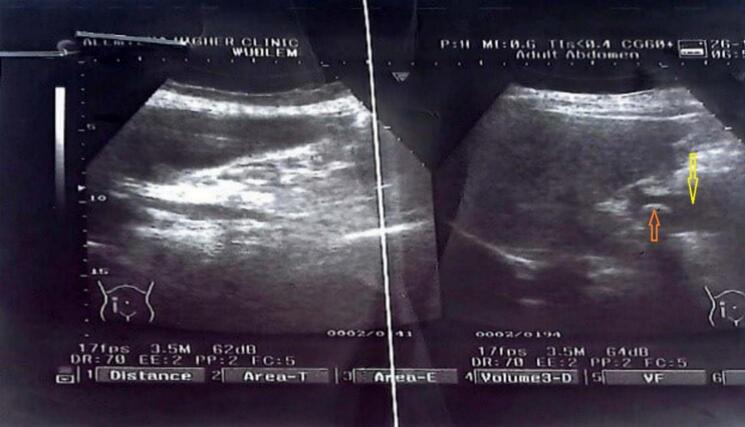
Preoperative ultrasound of a 40 years old female patient with a duplicated gallbladder. Yellow arrow showing anechoic structure indicating the gallbladder and Red arrow showing hyperechoic structure indicating the stone in the gallbladder. (For interpretation of the references to colour in this figure legend, the reader is referred to the web version of this article.)
